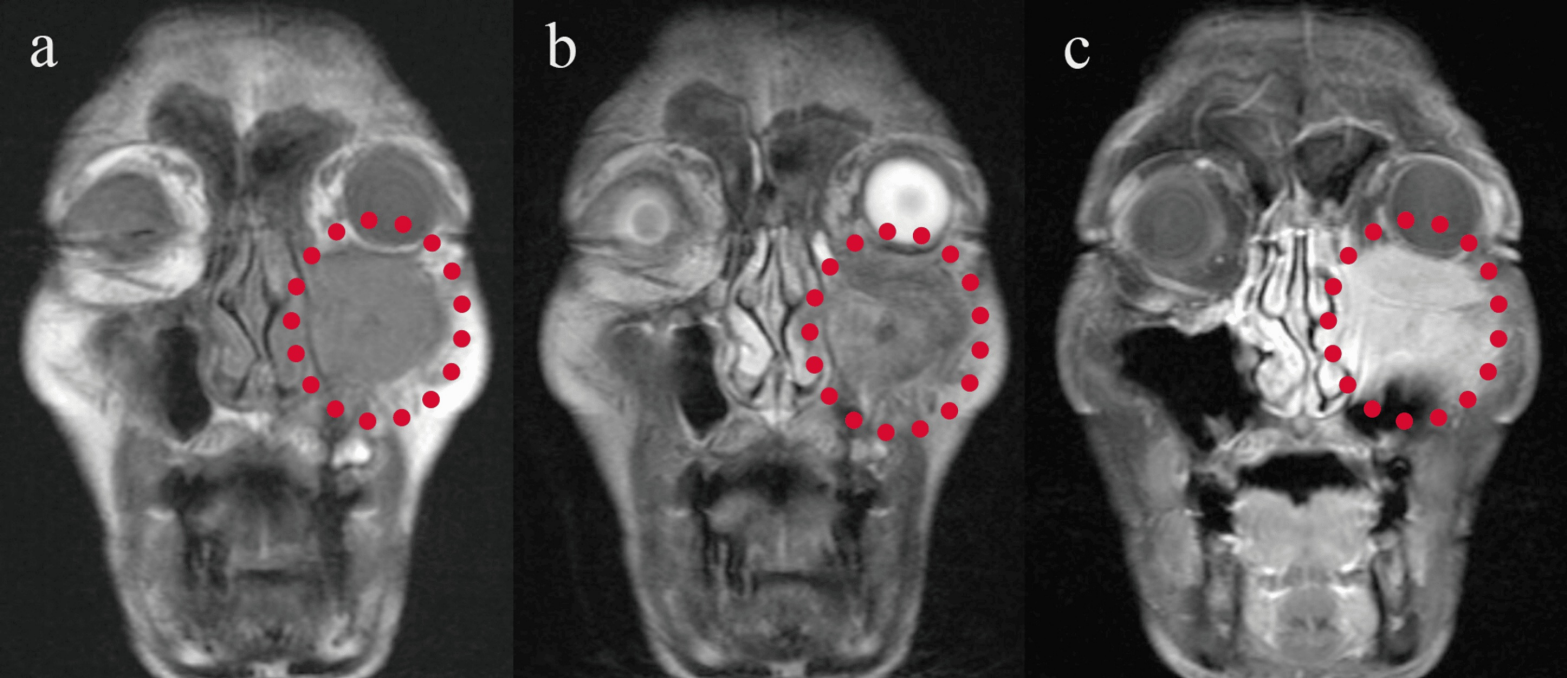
MRI images An abnormal lesion (circle area), with low signal on T1 (a) and high signal on T2 (b), is seen, and the contrast effect extends into the orbit on contrast T1 (c). MRI, magnetic resonance imaging
